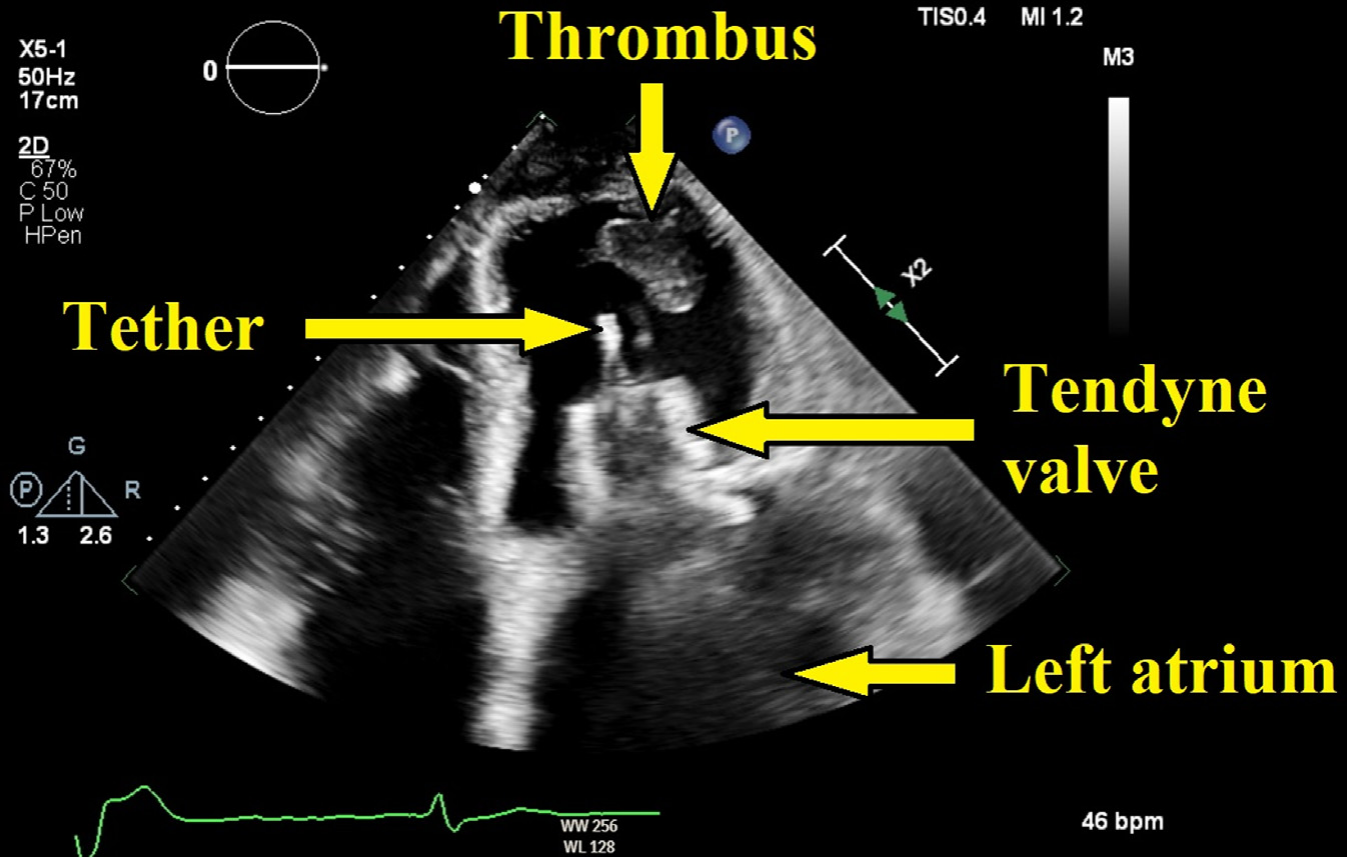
Transthoracic Echocardiogram in Apical 4-Chamber View Transthoracic echocardiogram in apical 4-chamber view (still image) showing the dual-frame transcatheter mitral valve replacement valve and its tether. Note the very large pedunculated mass attached to the apical end of the tether.
